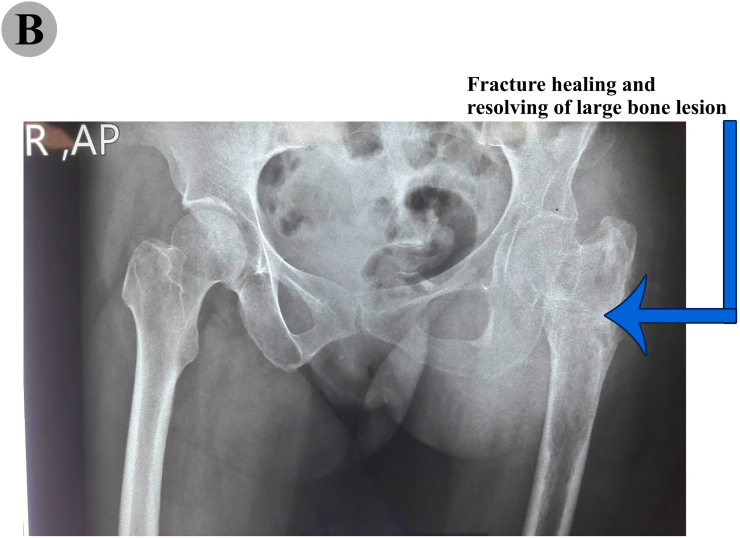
(A) T1/T2 weighted image of pelvis and left proximal part of the femur. The MRI scans showed a normal sub-periosteal space, without evidence of mass and no involvement of surrounded soft tissue and underlying cortex following treatment. 5. (B) An anteroposterior (AP) radiograph of the pelvic post combined treatment with chemotherapy and irradiation demonstrated fracture healing and no evidence of mass and involvement of surrounded soft tissues.
